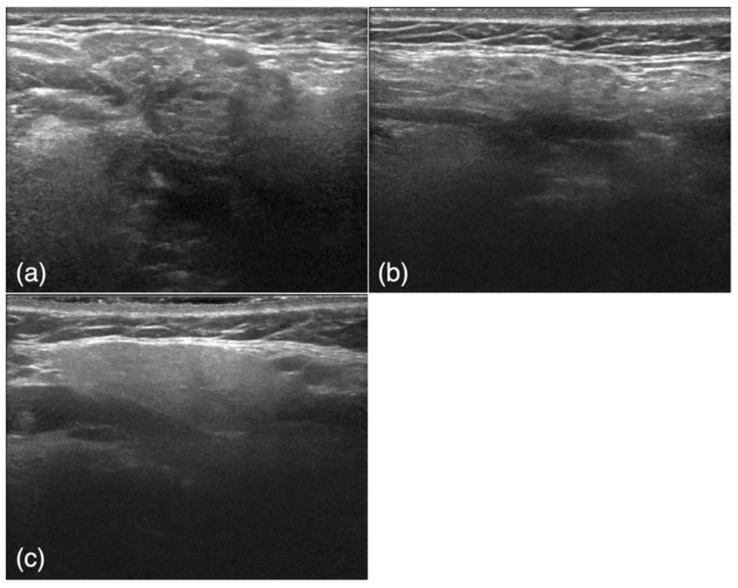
Examples of ultrasound images. (a) A patient in the obstructive sialoadenitis group shows inhomogeneous parenchyma and well-defined margins. (b) A patient in the Sjögren’s syndrome group shows inhomogeneous parenchyma characterized by multiple diffuse anechoic regions and ill-defined margins. (c) A patient in the control group shows homogeneous parenchyma and well-defined margins.
